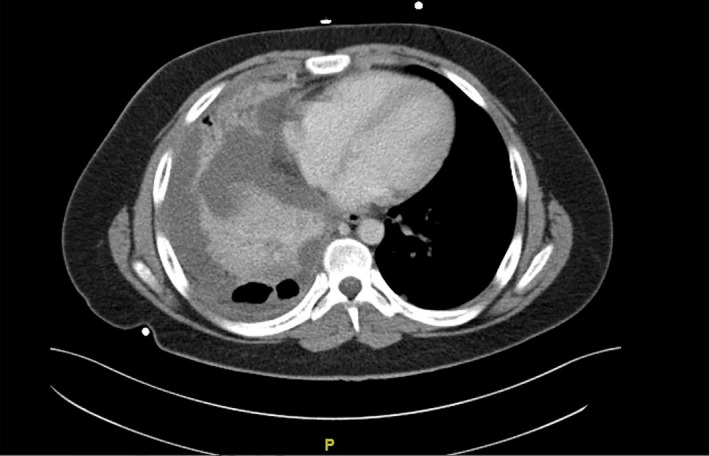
Mediastinal view of CT chest showing right‐sided pleural effusion
